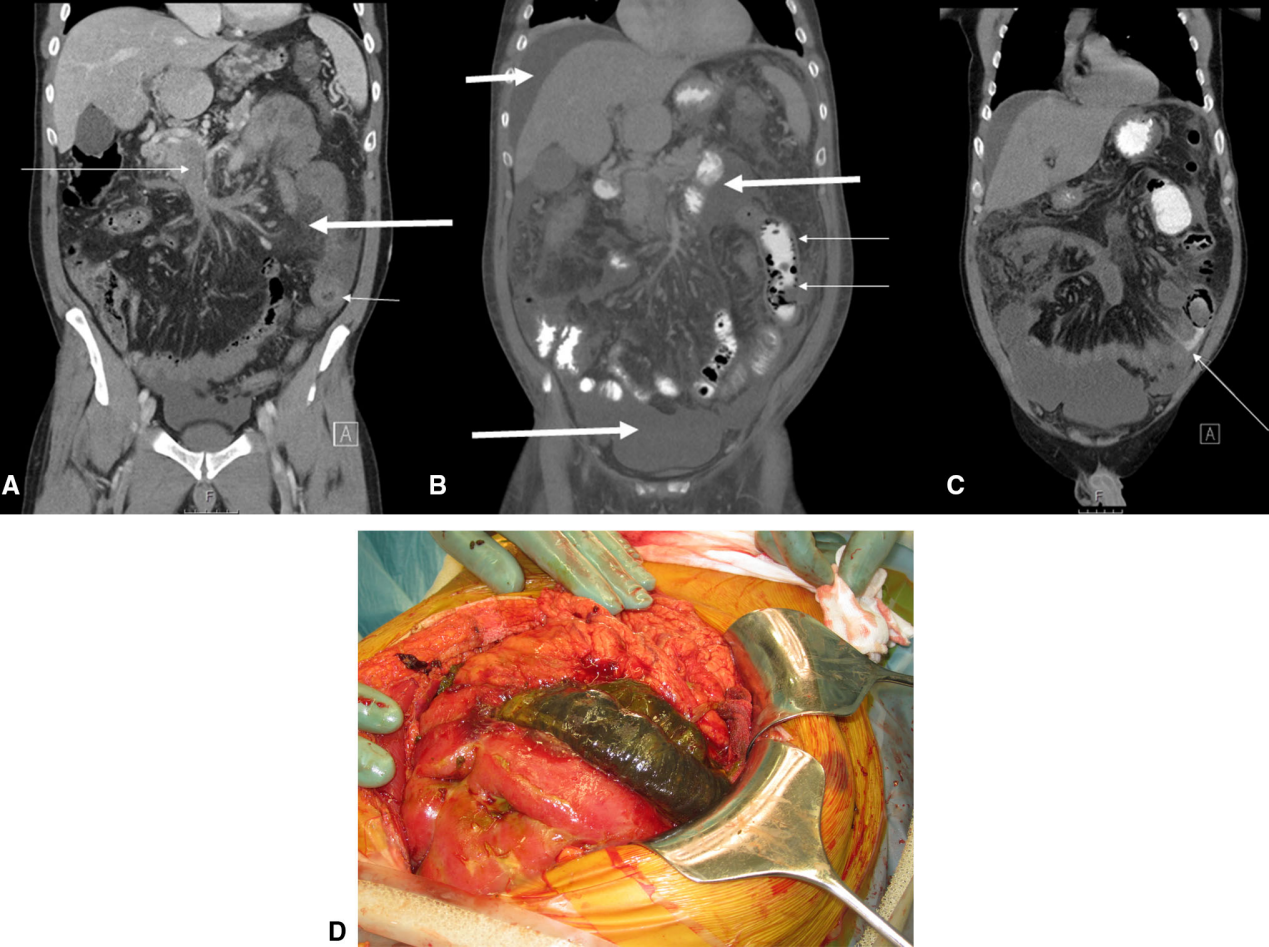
Failure of anticoagulation therapy. A 50-year-old male patient with a history of ulcerative proctitis who was admitted with 3 days of abdominal pain and C-reactive protein (CRP) of 161 mg/L. Diagnosis of mesenteric venous thrombosis (Fig. 2a, long thin arrow) was achieved after computed tomography (CT) with intravenous contrast enhancement and imaging in the portal/parenchymal phase. Note thickening of the jejunum (short arrow) and the mesenteric edema (long thick arrow). The patient had localized signs of peritonitis to the left in the abdomen and absent bowel sounds at the time of diagnosis. Full-dose heparin infusion was started, whereafter the patient improved temporarily but later deteriorated. A new CT (Fig. 2b) after 13 days of heparin therapy showed progression of ascites (thick arrows) and occurrence of gas bubbles (thin arrows) in the jejunal wall. Continued conservative therapy resulted in further clinical deterioration, and after 20 days of heparin therapy a CT (Fig. 2c) showed leakage of perorally administered contrast outside of the bowels (arrow). Explorative laparotomy showed a well-demarcated 1-meter-long transmural green necrosis of the jejunum (Fig. 2d) with a large perforation. The patient recovered after bowel resection, open abdomen therapy, and reanastomosis of the stapled bowel ends. Testing for thrombophilia showed that the patient was positive for JAK2 V617F mutation and a bone marrow biopsy diagnosed a polycythemia vera. The patient is scheduled for lifelong vitamin K antagonist therapy, and cytoreductive therapy with interferon, and is also undergoing regular venesection
